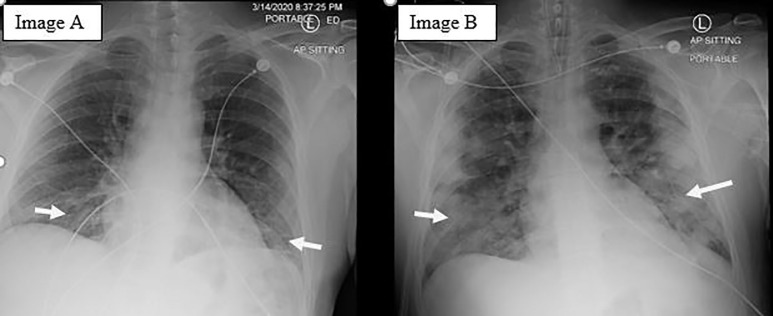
A) Portable chest radiograph on presentation to the emergency department, demonstrating subtle, patchy infiltrates visible in the lung bases suggesting early pneumonia (arrows). B) An anterior posterior chest radiograph two days after admission, demonstrating significant interval progression of peripherally located patchy opacities throughout both lungs (arrows) with areas of consolidation at the lung bases and right upper lobe.
